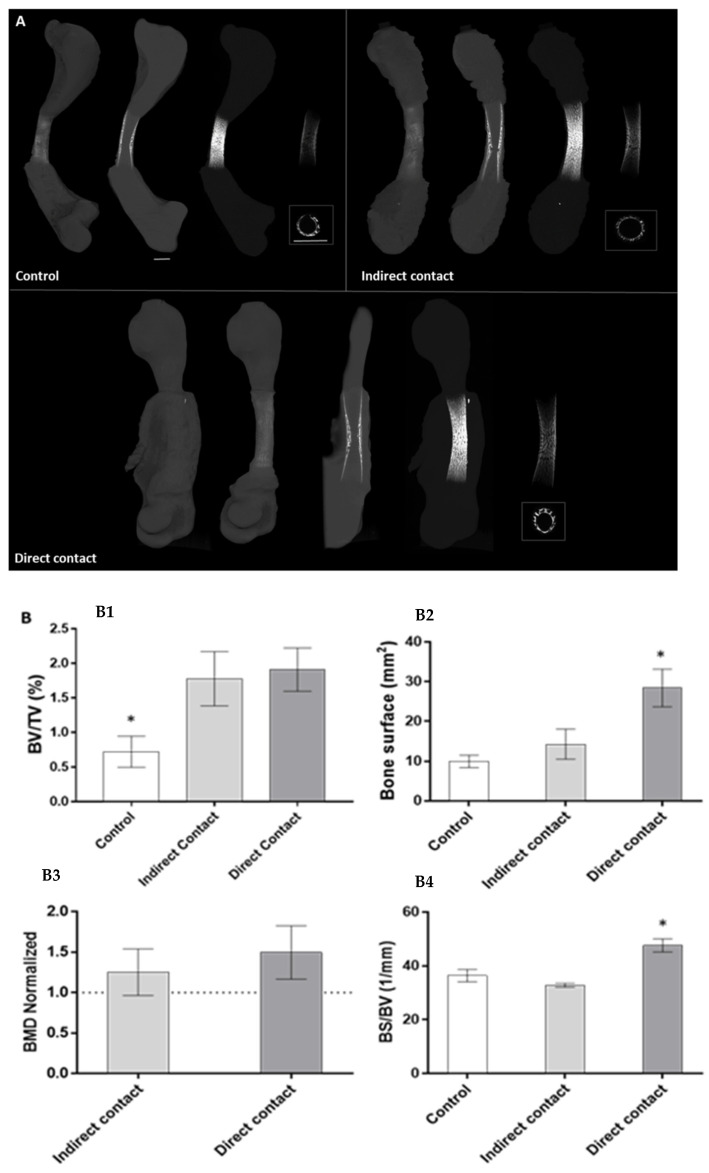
Microcomputed tomography analysis of D11 chick femora following 11-day culture in the absence (control) or presence of L-PRF membranes, in indirect and direct contact. (A)—Three-dimensional representative images of samples (whole femur, sagittal section and maximum intensity projection of the whole femur, sagittal/ cross-section of the central diaphysis region, respectively) of the distinct experimental conditions. Scale bar corresponds to 500 µm. (B)—Quantitative morphometric parameters of the samples: (B1)—BV/TV; (B2)—BS; (B3)—BMD; (B4)—BS/BV). BMD was normalized to the control values, corresponding to 1 unit. * Statistically significant, *p* < 0.05, *n* = 6.
